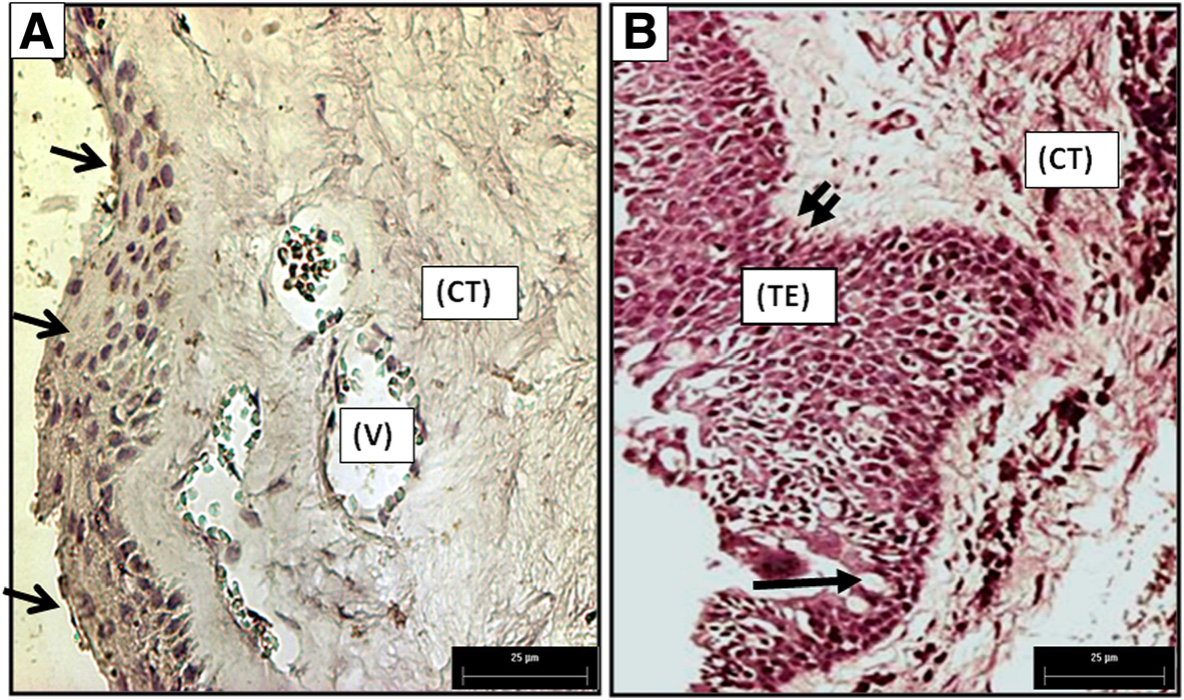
(A) A healthy conjunctiva lacking evidence of inflammatory cell infiltration, containing few vessels (V), normal epithelium (arrows), and uniform connective tissue (CT). (B) Primary Pterygium. Thickened epithelium (TE), epithelial goblet cells (arrow), and basal lamina (double arrow), on its right, we identified connective tissue (CT) (400× Magnification).
